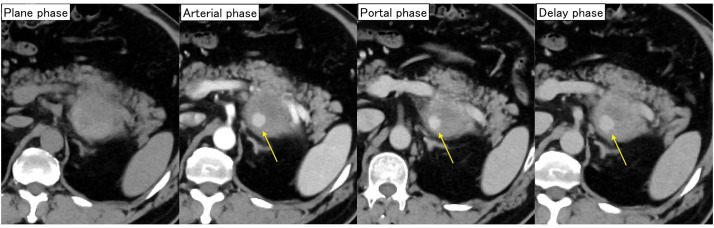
CT scan findings in the previous hospital. A dynamic-enhanced CT scan revealed a 60-mm hypovascular mass on the dorsal side of the pancreas and extravasation inside the mass (arrows).
